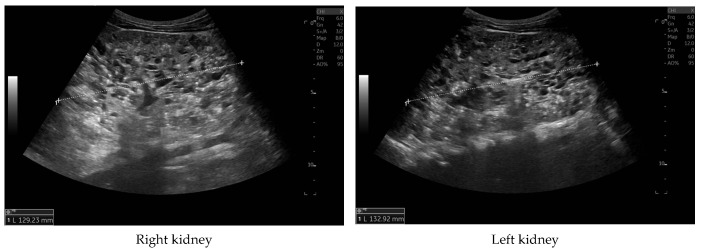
Ultrasonography of kidneys at the age of five years.
